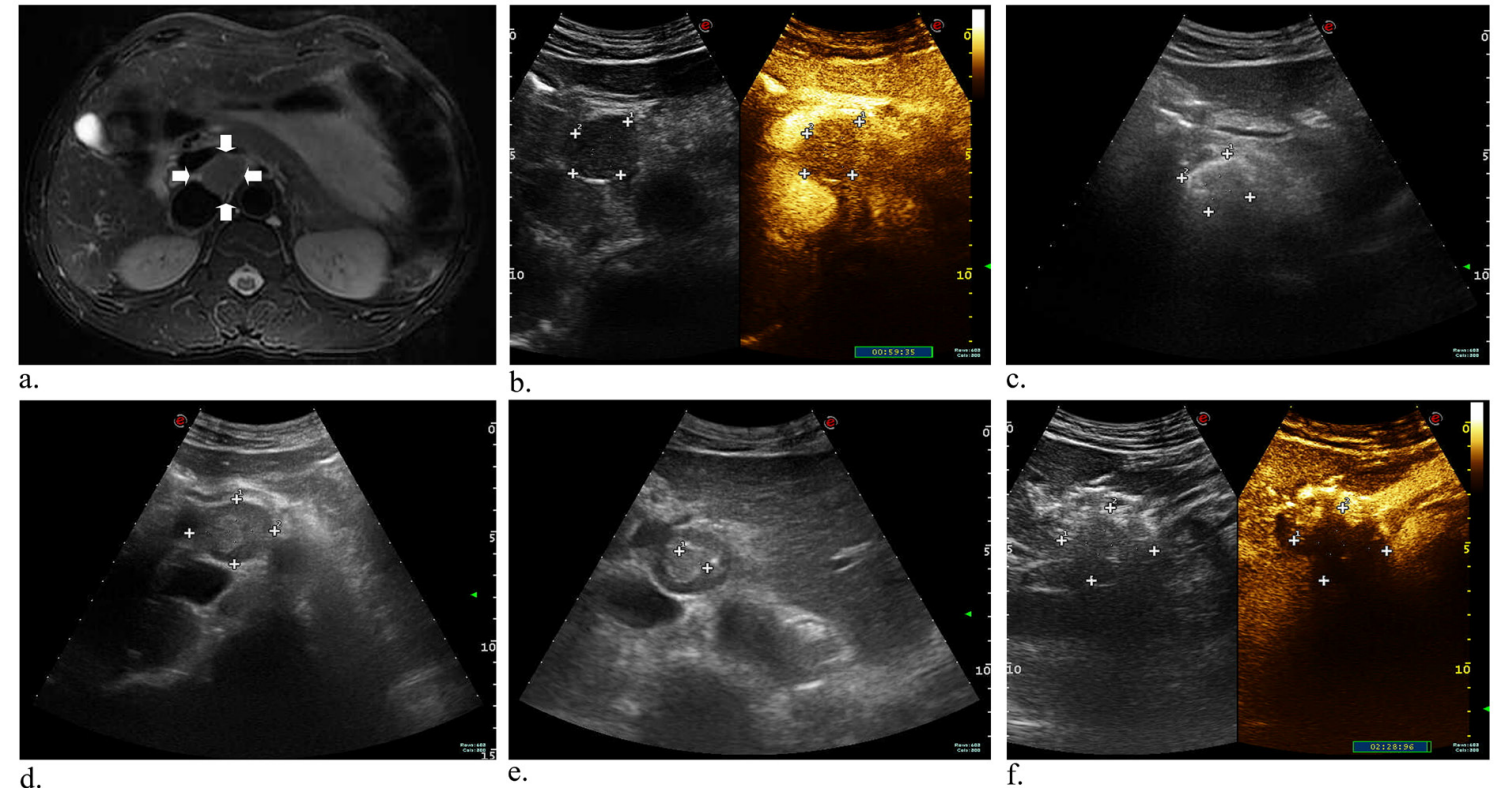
A 43-year-old man with metastatic lymph nodes originating from liver cancer Axial contrast-enhanced MR image was obtained at the abdominal setting. An oval, mildly high signal intensity is present close to aorta abdominalis a. (arrowhead). Preoperative CEUS images showed the lesion with rapid wash-in and wash-out in arterial b.. Axial gray-scale US image indicated intraoperative ethanol ablation c.. Before initial laser ablation, the mass is shown in the retroperitoneum under US guidance d.. Two laser fibers parallelly ablated the tumor under the guidance of US, which appraised the distances (cm) of needle tip to mass boundary e. (anterior: 1.9; 1.8; left: 0.9; right: 0.7), and subsequent immediate CEUS image showing a large and central filling defect f..
